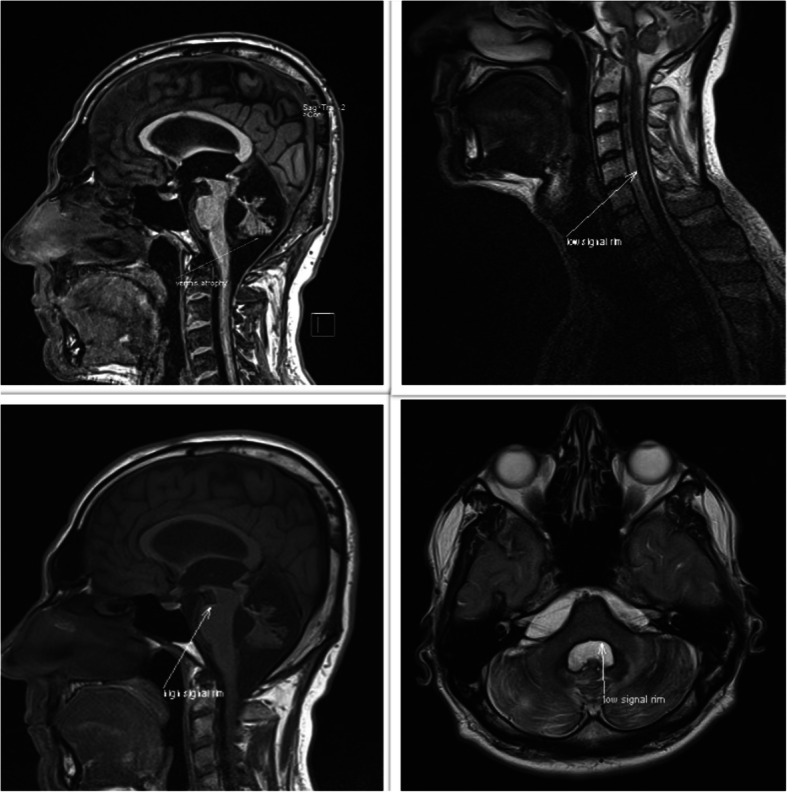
MRI brain images of the patient 2 showing features characteristic of superficial siderosis. A rim of low intensity coating the surface of the brain and spinal cord with atrophy of cerebellar vermis
